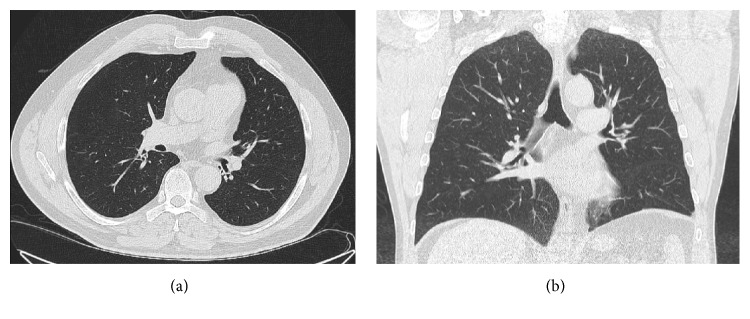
CT scan of the chest (lung window) in horizontal (a) and coronal (b) sections showing a normal lung parenchyma with complete resolution of the left lower lobe infiltrate noted in Figure 1.
